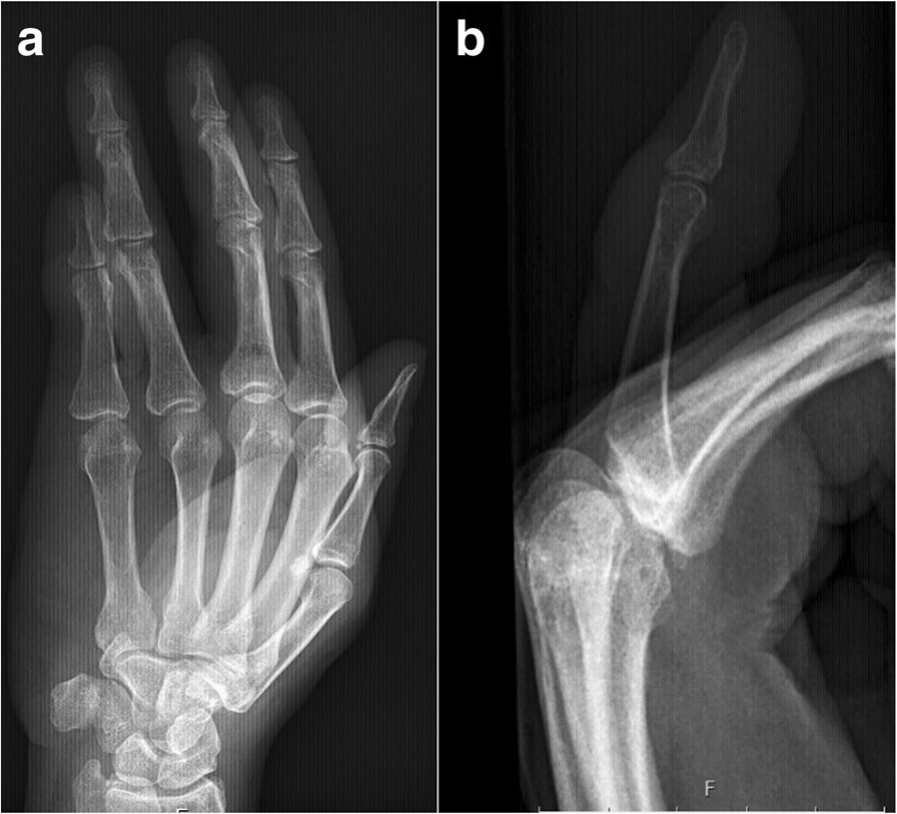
Radiograph of patient 1 showing (a) posteroanterior and (b) lateral views of the small finger
